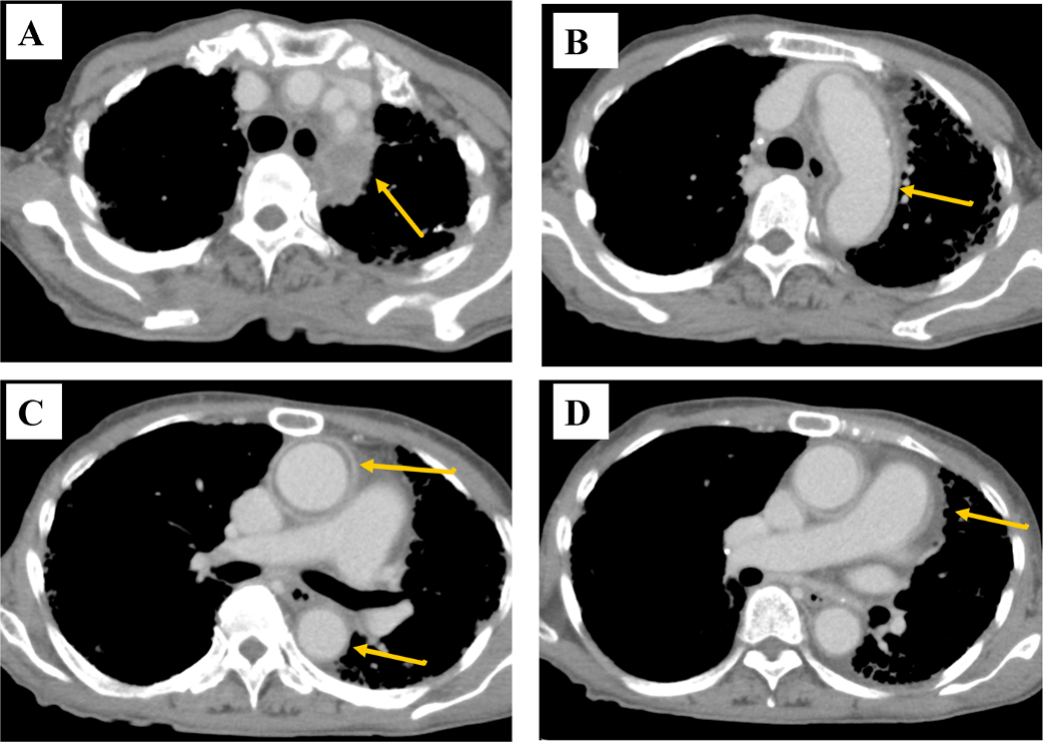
Thoracic contrast enhanced CT findings of aortitis before steroid therapy. Thoracic contrast-enhanced CT demonstrated thickening of the aortic wall at each following level with a contrast effect, and the non-contrast layered area on the lumen. A. brachiocephalic artery and left subclavian artery. B. aortic arch. C and D. ascending aorta.
